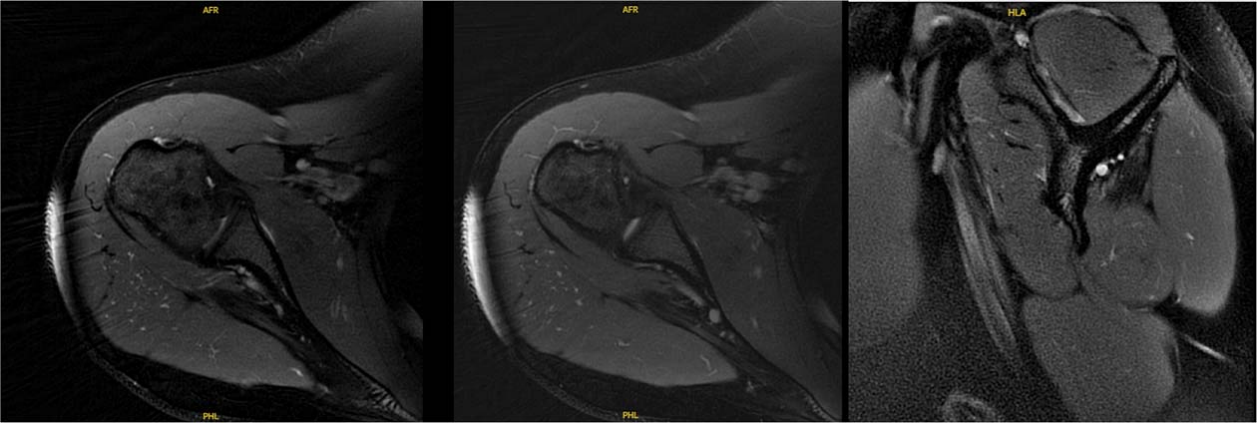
MRI 6 years after decompression revealed a diffusely atrophic infraspinatus muscle belly.
